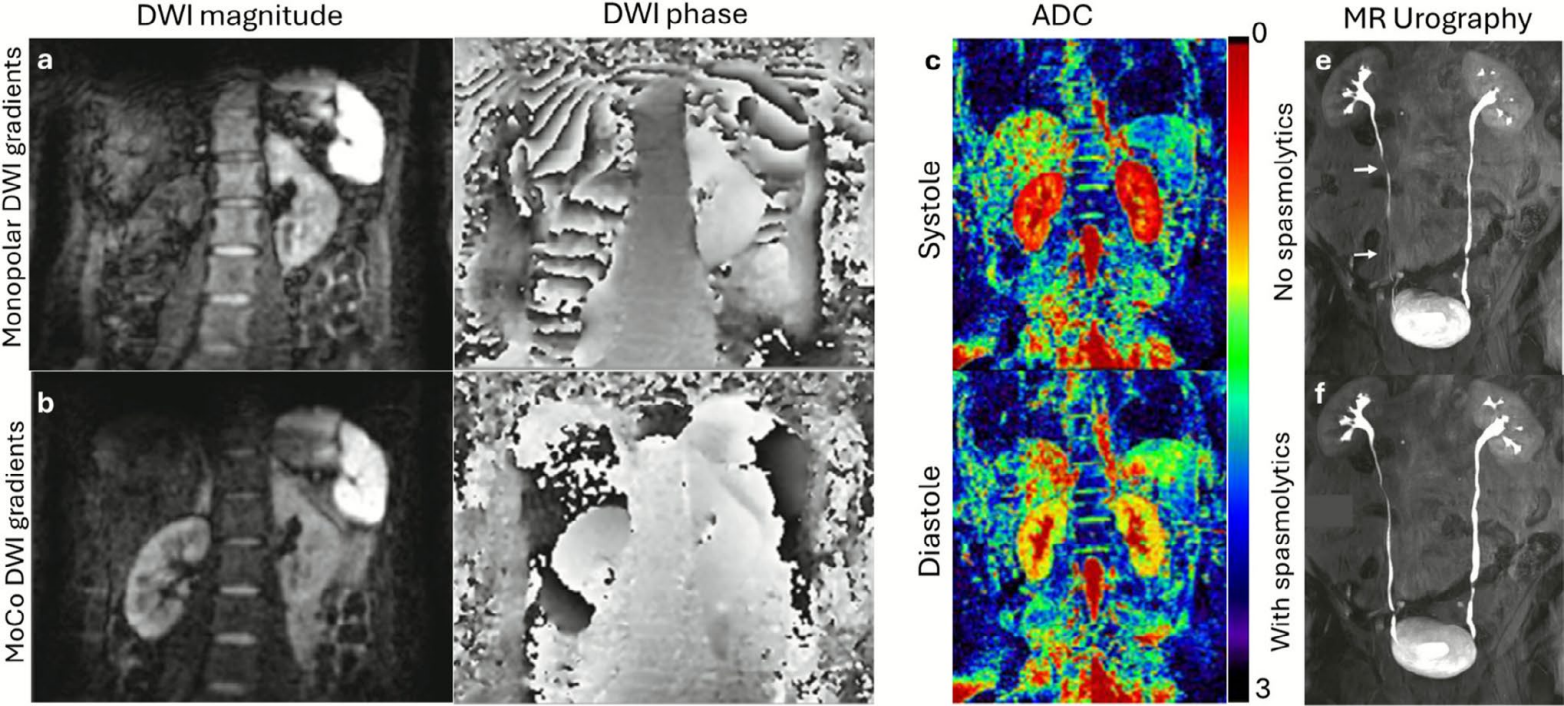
Respiratory, cardiac, and peristaltic motion effects in kidney MRI. (a and b) Renal examples of signal void and phase variability induced by motion in diffusion weighted imaging (DWI), along with a prospective approach using velocity compensated diffusion gradients that reduces these effects. (c and d) As the kidneys are highly perfused, apparent diffusion coefficient (ADC) maps display cardiac pulsation‐induced variability. (e and f) Motion artifacts from ureter peristalsis can be mitigated through the use of spasmolytics. Adapted from [68, 76, 77].
